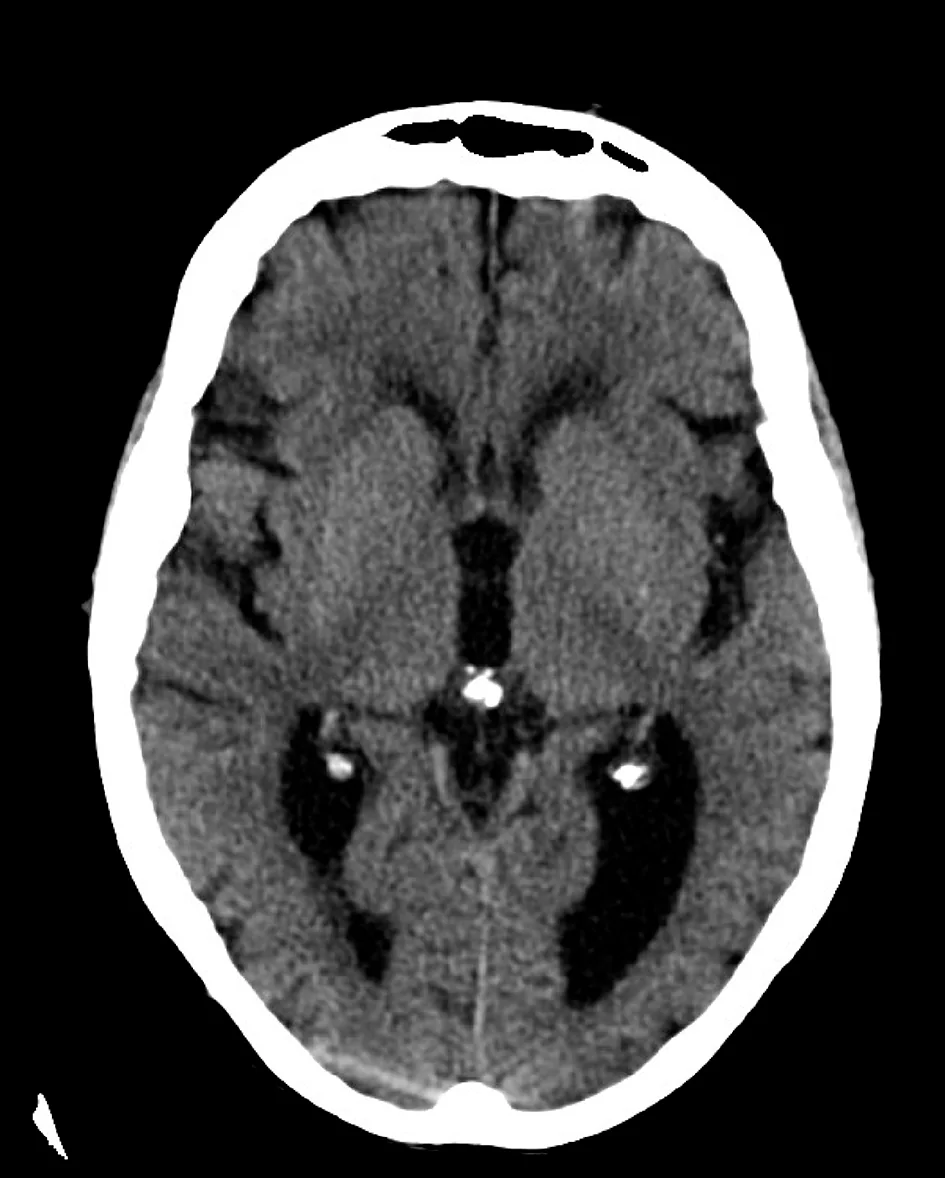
Computed tomography of head revealed no cerebral embolism
